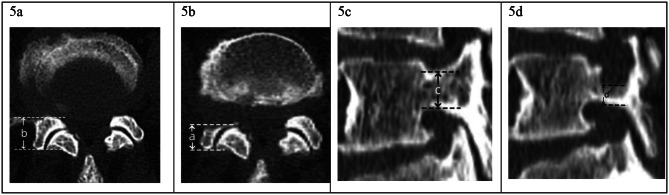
Calculation of the resection range rate of facet joint and pedicle. (a), (b): Resection range rate measured on axial CT image obtained before (left) and immediately after the surgery (right), the equation is (b-a)/b; (c), (d): Resection range rate measured on sagittal CT image obtained before (left) and immediately after the surgery (right), the equation is (c–d)/c
